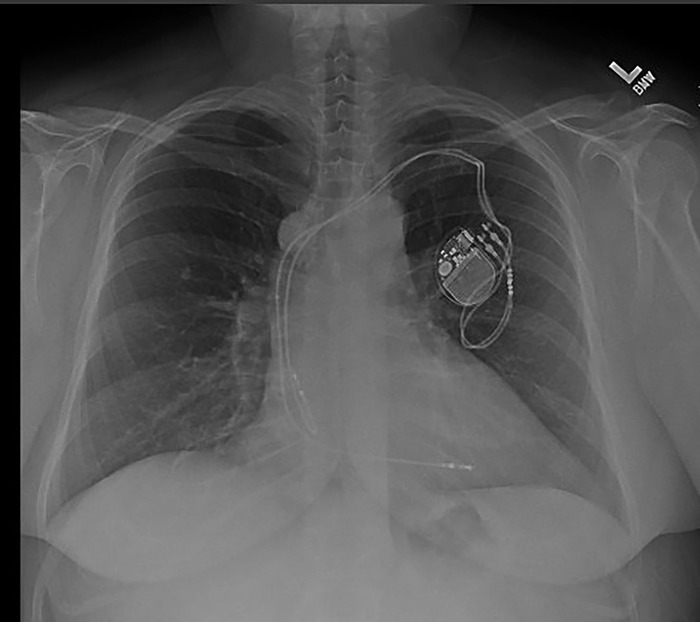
Preoperative chest x-ray shows a calcified mass—the bulge to the left of the aorta near the superior vena cava—in the right paratracheal area.
